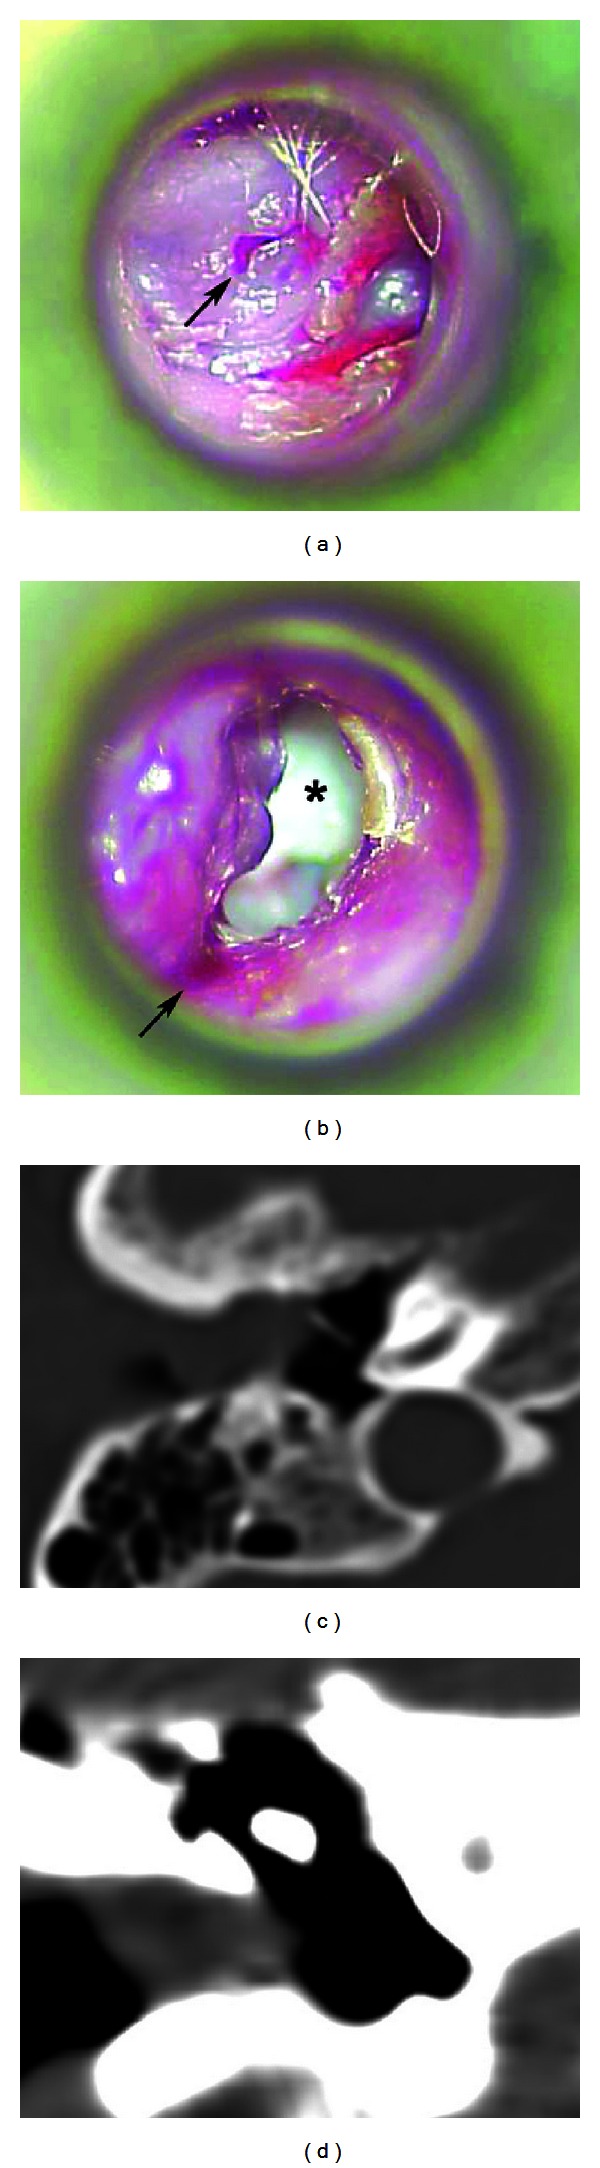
(a) Macroscopic appearance of the EAC polypoid mass with a hole in the center (arrow). (b) When the tumor was elevated, granular tissue was observed at the inferior wall of the EAC (arrow). A large plug of desquamated keratin was observed behind the lesion (asterisk). (c) The soft-tissue mass obstructing the slightly widened bony part of the EAC. (d) A soft-tissue window CT scan revealed the nonhomogenous structure of the tumor.
